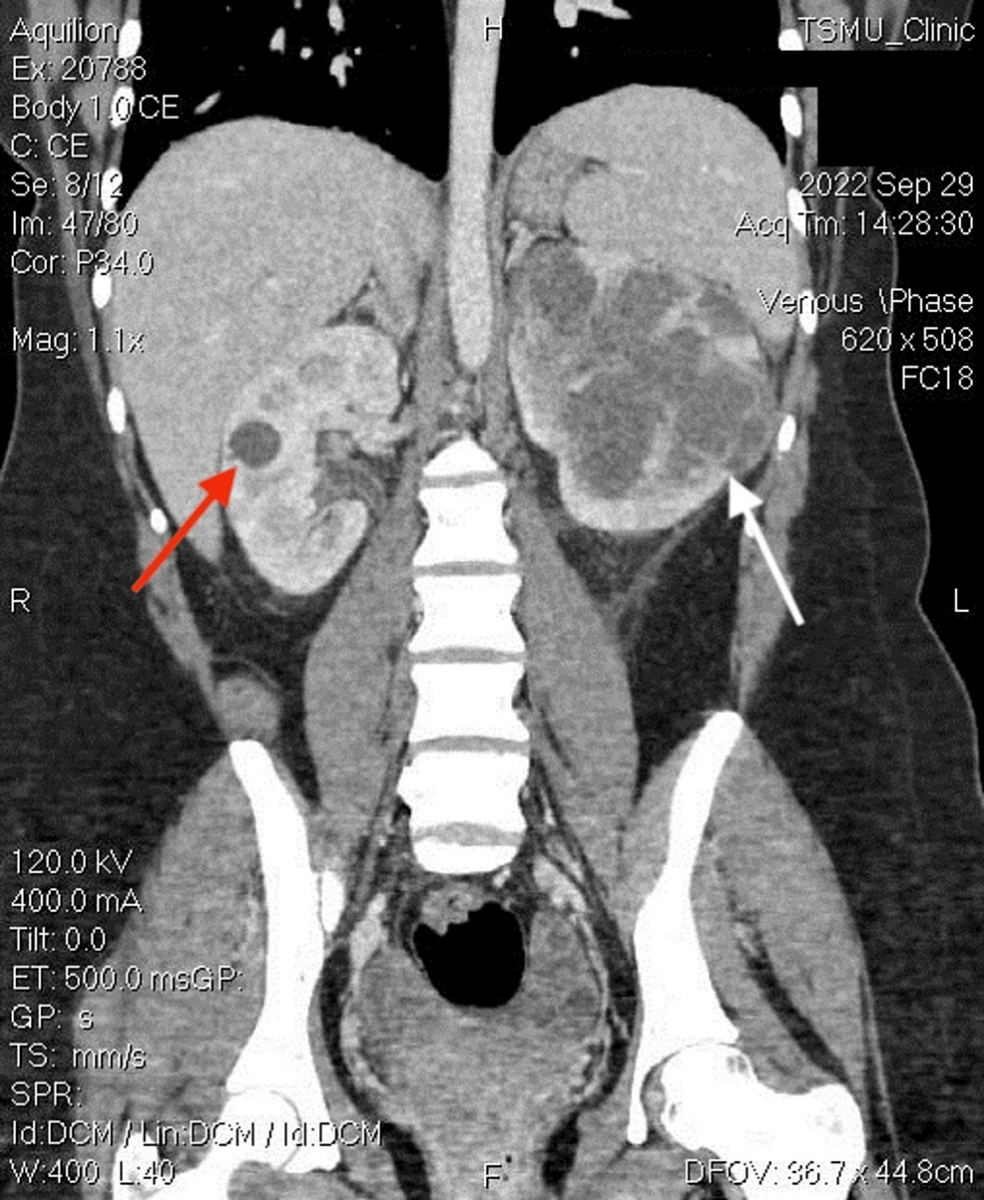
Coronal view of CT scan showing left-sided loculated renal abscess (white arrow) and right-sided renal cyst (red arrow). White arrow: left-sided loculated renal abscess. Red arrow: right-sided renal cyst. CT: computed tomography.
